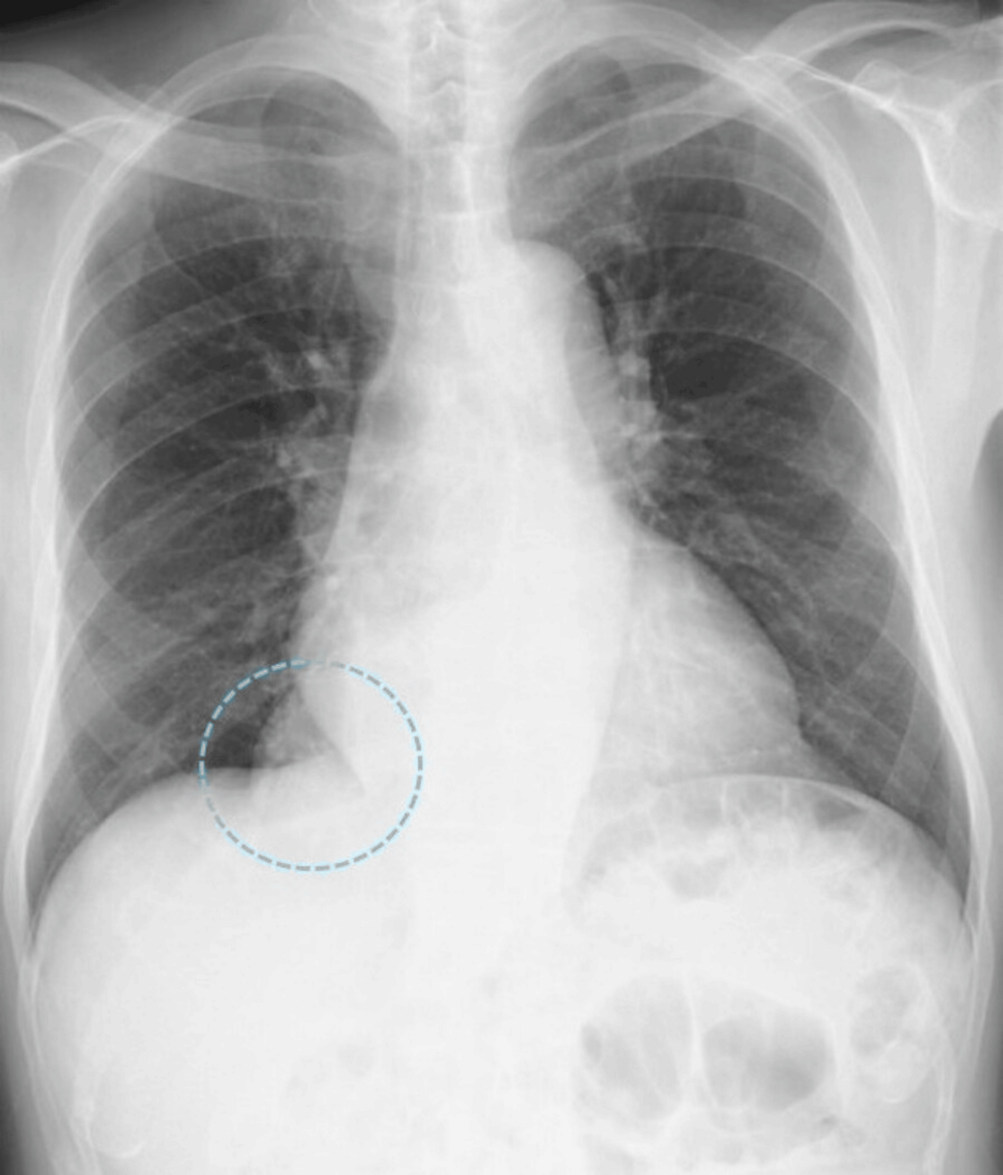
Chest X-ray Abnormal mediastinal shadows were noted, and a mediastinal tumor was suspected
